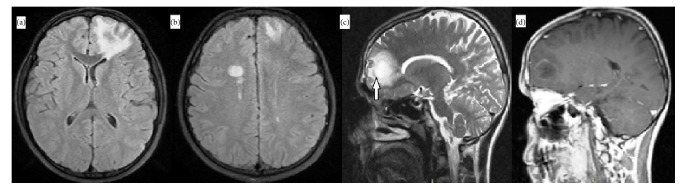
Initial imaging findings. (a) Axial fluid attenuation inversion recovery (FLAIR) image reveals a large hyperintense lesion in the left frontal lobe with surrounding vasogenic edema. (b) Axial FLAIR image shows hyperintense demyelinating lesions in bilateral subcortical white matter. (c) Sagittal T2-weighted image demonstrates T2 hypointense rim (arrow). (d) Sagittal T1-weighted postcontrast image shows ring enhancement.
